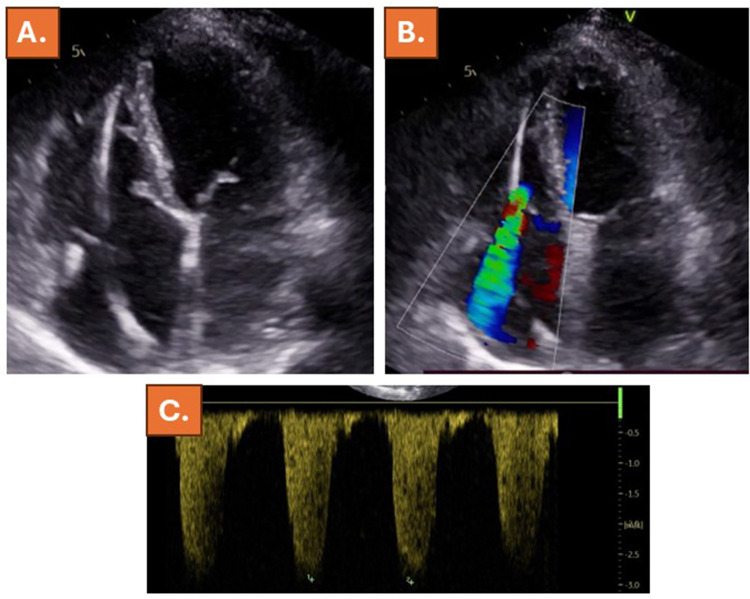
Four weeks after successful electric cardioversion, echocardiography revealed normalization of RV-diameters and function (A) and only mild- to moderate tricuspid regurgitation (B) with slightly elevated systolic pulmonary artery pressure of 40mmHg (C).
